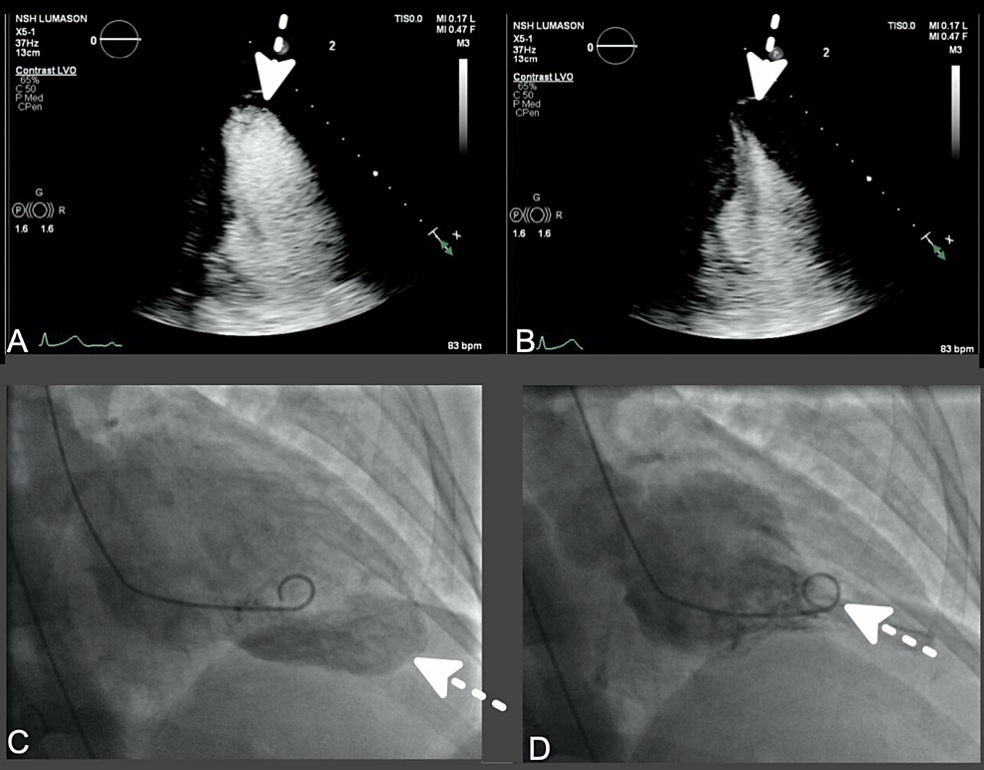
TTE four-chamber view with the use of definity echocardiography contrast at end-diastole (A) and end-systole (B). Left ventriculograms in end-diastole (C) and end-systole (D). Arrows point towards the cardiac apex. TTE: transthoracic echocardiogram
